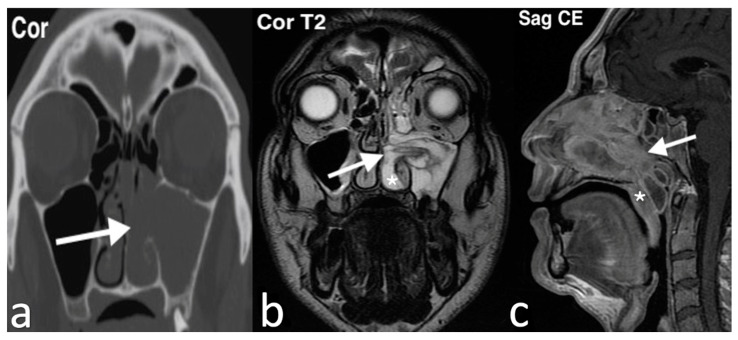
Antrochoanal polyp in a patient with left nasal obstruction and suspected rhinosinusitis. At rhinoscopy, a yellowish translucent lesion, arising from left ostiomeatal complex and extending up to the nasopharynx, is observed. The coronal CT image (a) shows a soft tissue mass filling the lumen of the left maxillary sinus and extending through an accessory ostium of the medial sinus wall in the adjacent nasal cavity (arrow); no evident bony erosion is visible. The MR coronal T2 image (b) shows a polypoid mass (arrow) with high intensity in the left maxillary sinus, extending through the middle meatus into the left nasal cavity between the inferior turbinate (asterisk), laterally displaced, and septum. Sagittal T1 after intravenous Gd image (c) shows peripheral enhancement of the mass (arrow), extending posteriorly toward the choana in the nasopharynx lumen, behind the soft palate (asterisk); note the mucosal thickening of sphenoid and frontal sinuses due to obstruction of ostiomeatal complex.
